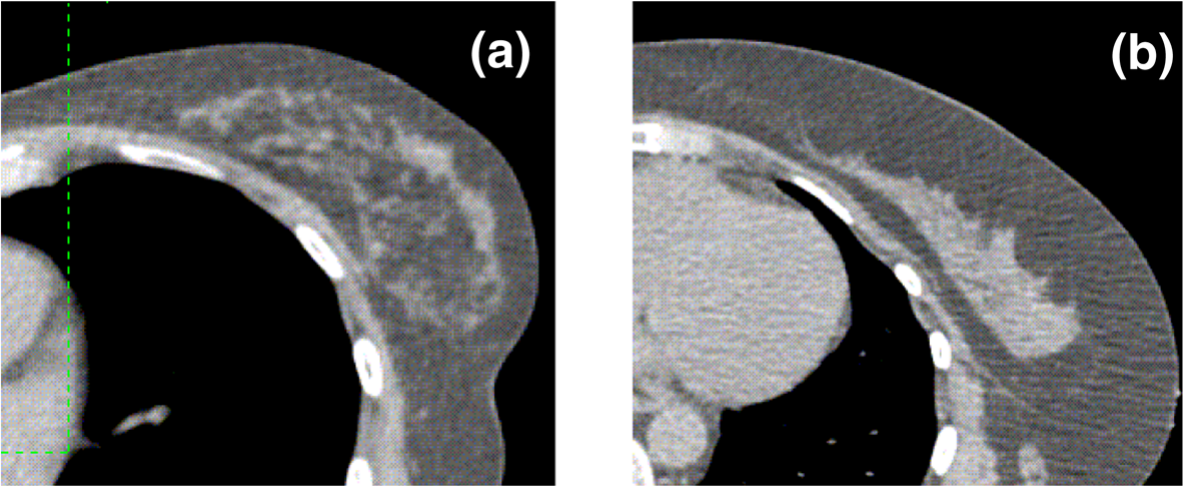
Sample fibroglandular distributions in the breast, middle-breast CT images: a Breast with sparse distribution of fibroglandular tissue, b Breast with non-spare distribution of fibroglandular tissue
